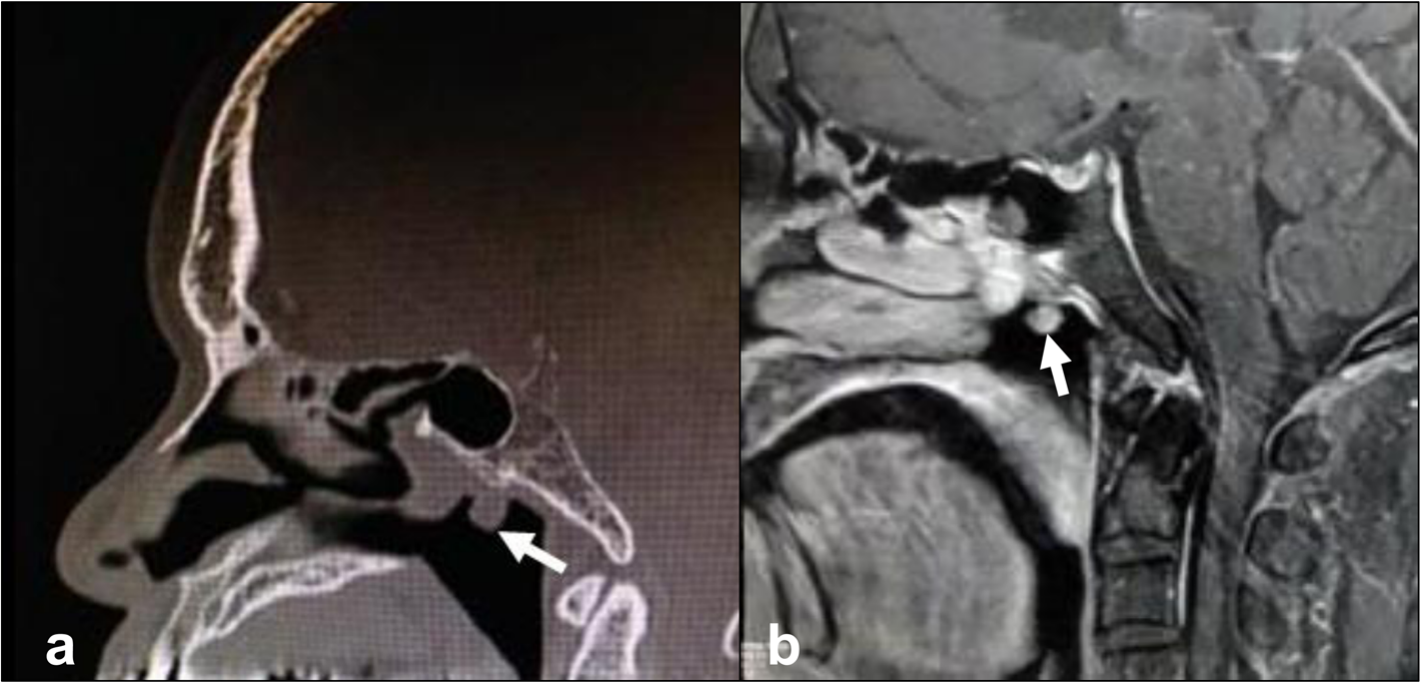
Tumor imaging. a Computed tomography image (no enhancement) and (b) magnetic resonance image (T1-weighted image gadolinium positive). White arrows show the tumor
